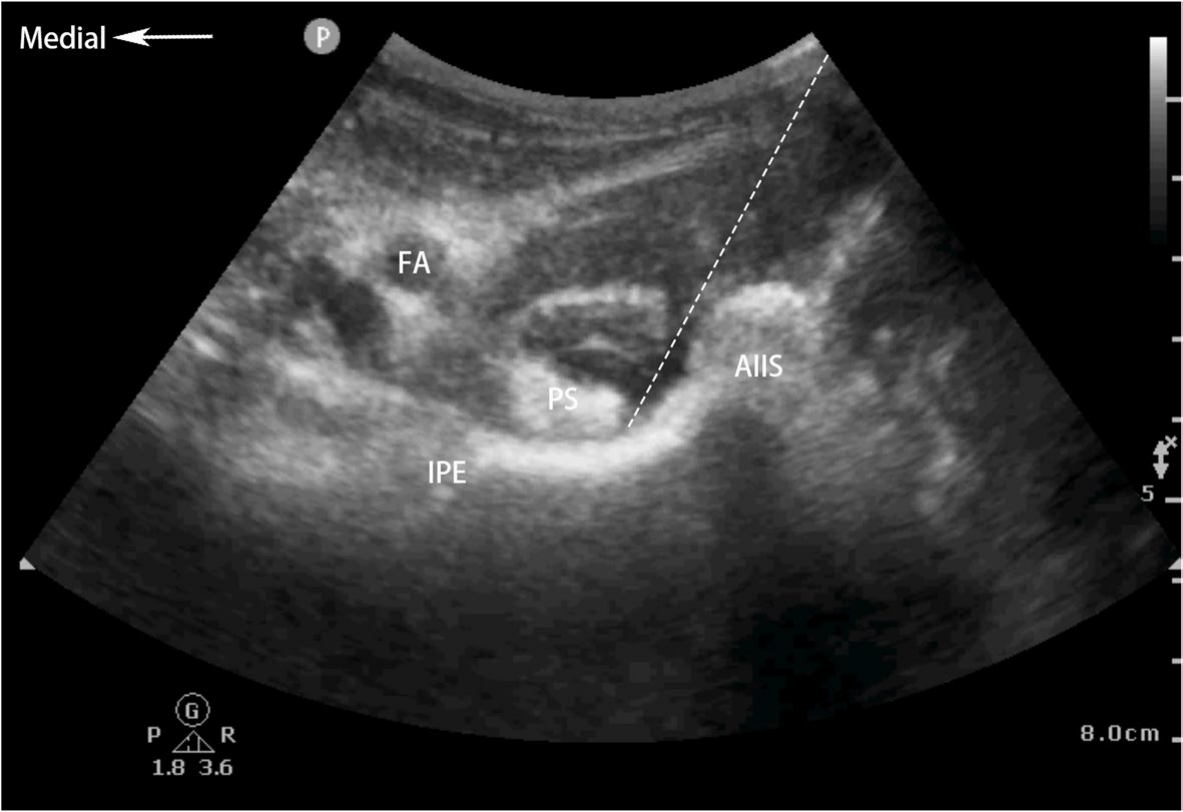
Ultrasound imaging of SIFIB. Dashed line indicates the fascia iliaca; ASIS, anterior superior iliac spine; IM, iliacus muscle; IO, internal oblique; IP, iliopsoas muscle; and TA, transverse abdominus
